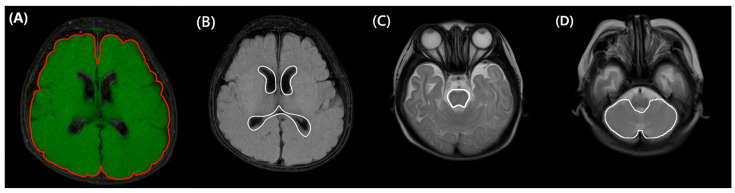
Regional structure marking: Intracranial (A) and ventricle (B) marking was drawn on an axial FLAIR T2 FAT sequence. Brain stem (C) and cerebellar (D) marking was drawn on a T2-Weighted TSE sequence. FLAIR, Fluid-Attenuated Inversion Recovery.
